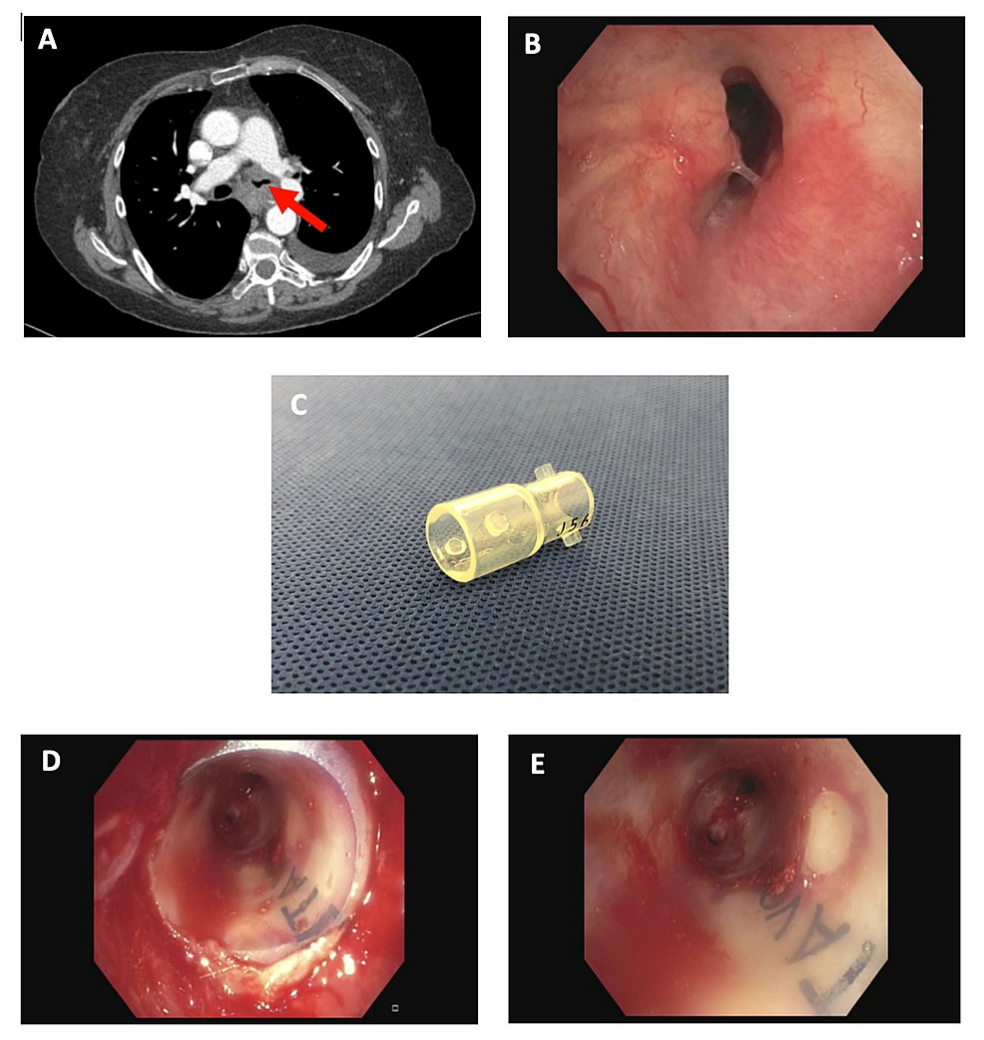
Left mainstem bronchial obstruction treated with modified hourglass stent A: CT demonstrating LMSB obstruction (arrow). B: Bronchoscopic image of LMSB obstruction. C: Modified hourglass stent. D: Post-stent insertion into the LMSB, proximal end. E: Post-stent insertion into the LMSB, distal end CT: computed tomography; LMSB: left mainstem bronchus
